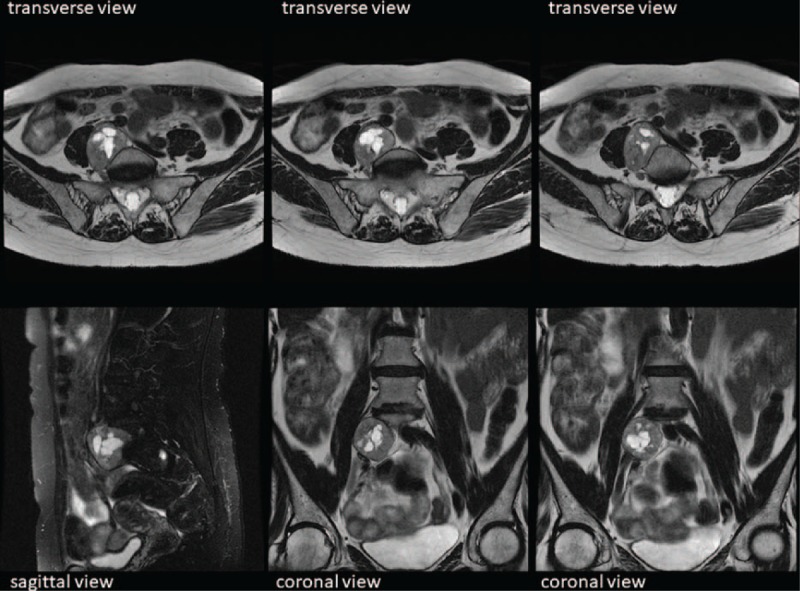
Magnetic resonance imaging. Transverse, sagittal, and coronal scan showing a well-demarcated retroperitoneal mass anterior to the soma of the 5^th^ lumbar vertebra, attached to the inferior vena cava and the right common iliac vein, and anteriorly dislocating the ipsilateral iliac arterial axis.
